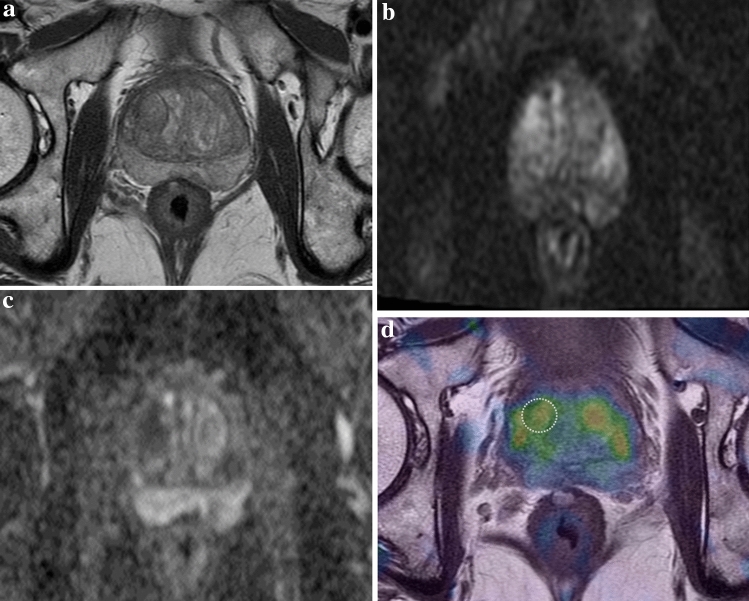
A 75-year-old man with benign prostatic hyperplasia confirmed using MRI-US fusion targeted biopsy. (a) T2-weighted axial MR image showing a well-encapsulated hypointense nodule in the right mid-transitional zone of the prostate. (b, c) Diffusion-weighted image (b = 1500 s/mm^2^) and ADC map show diffusion restriction in the corresponding area (ADC value, 0.735 × 10^−3^m^2^/s). (d) Elastogram shows that the mean stiffness of the tumor is 3.982 kPa.
